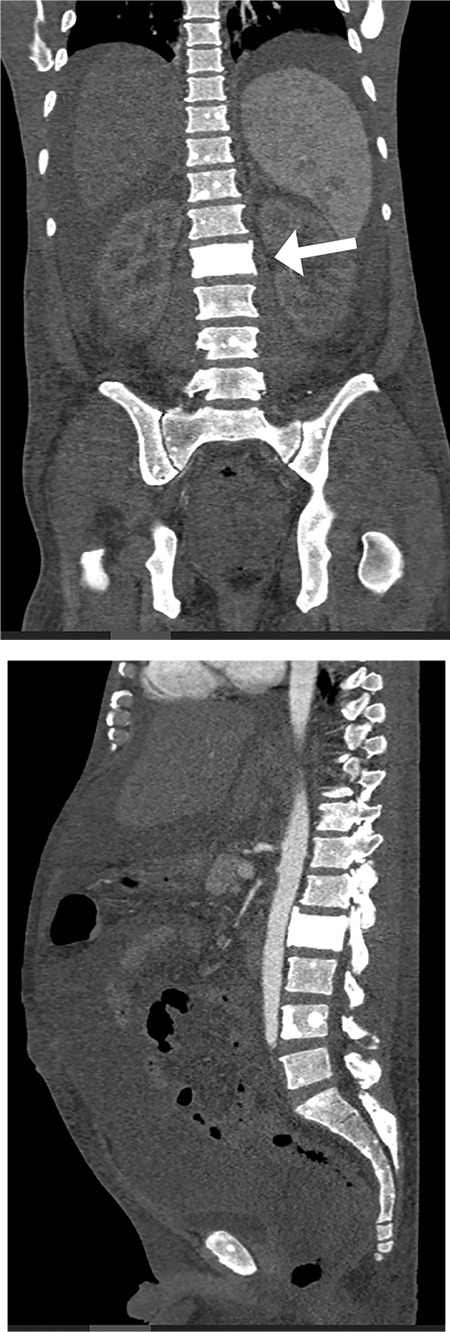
Computed tomography scan of the abdomen and pelvis showing multiple sclerotic bony lesions over the spine with ivory vertebral appearance of L1 (white arrow)
